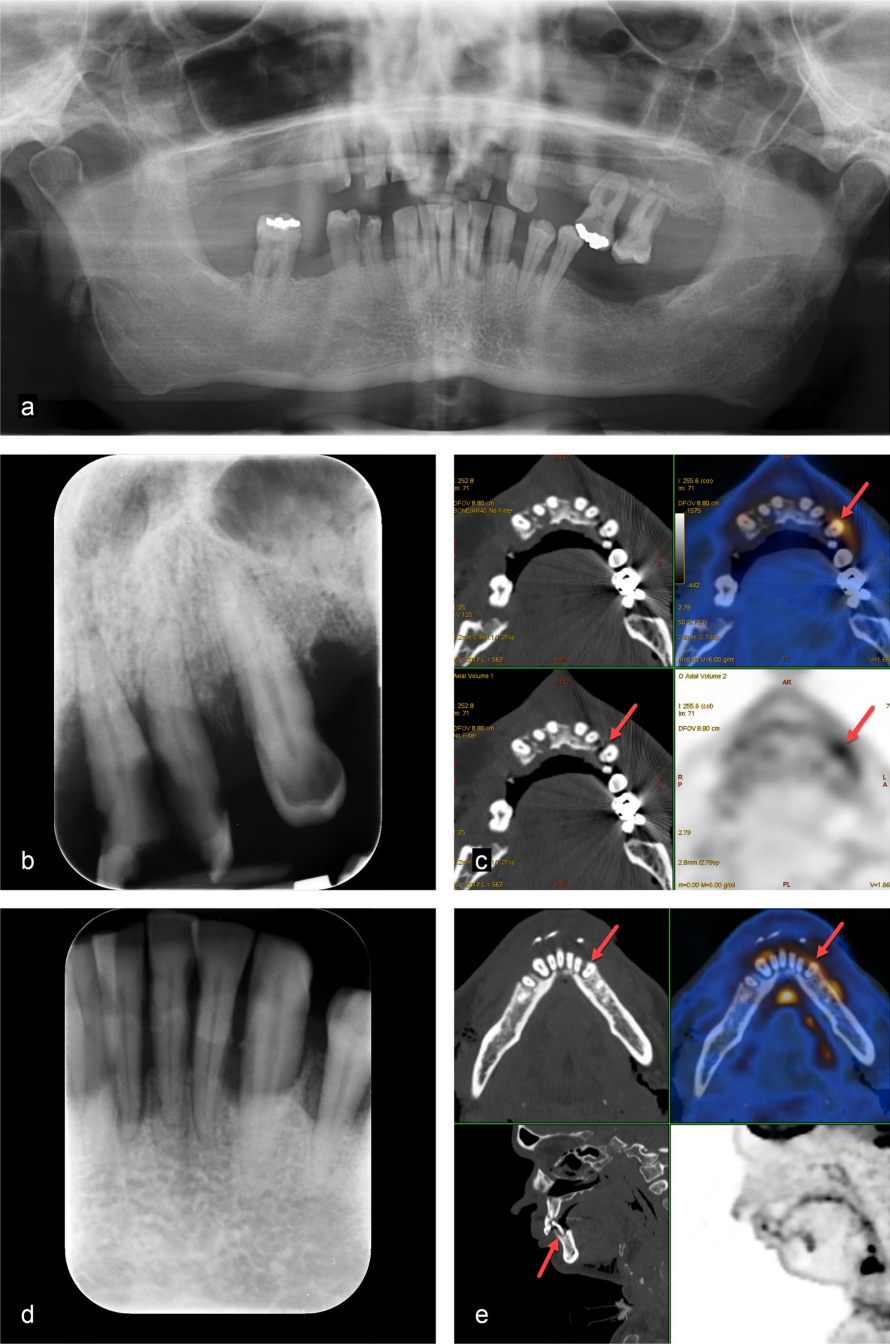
A 60-year-old man with glottic larynx carcinoma cT3 cN2c cM0. Clinical examination showed a generalised, severe chronic periodontitis and heavily decayed teeth due to caries and poor oral hygiene. Two out of several metabolically active pathologies are presented here in more detail. a Panoramic radiography gives an overview on the general periodontal situation. b, d The periapical radiographs of the upper left maxilla and the mandibular front show marginal periodontitis and vertical alveolar defects as well as tooth substance decay. c, e FDG-PET/CT demonstrates five tooth-related pathologies as metabolically active. The metabolic activity outlines the extent of the periodontal defects reaching to the apex for tooth 32 with a periapical periodontitis (SUV_max_ 6.6 for tooth 23 and SUV_max_ 4.6 for tooth 32, red arrows)
